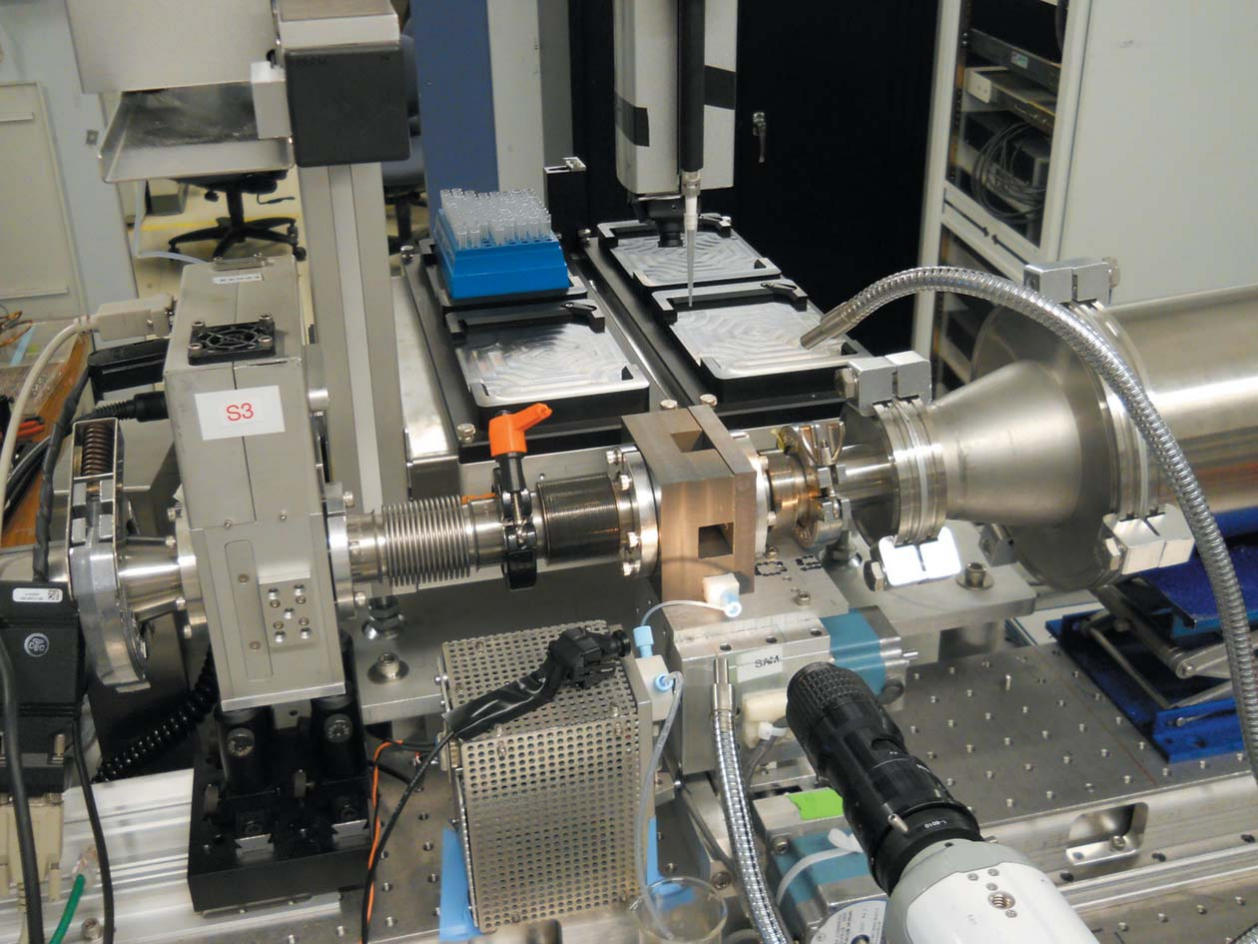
The BioSAXS set-up, shown here at CHESS G1 line, includes a quartz capillary flow cell housing (block at center) with a port at the top through which the robotic pipetting system (top) loads a sample. The X-ray beam travels entirely through vacuum from left to right in this figure. The video camera (front) provides a transverse *in vacuo* view of the flow cell for sample alignment.
